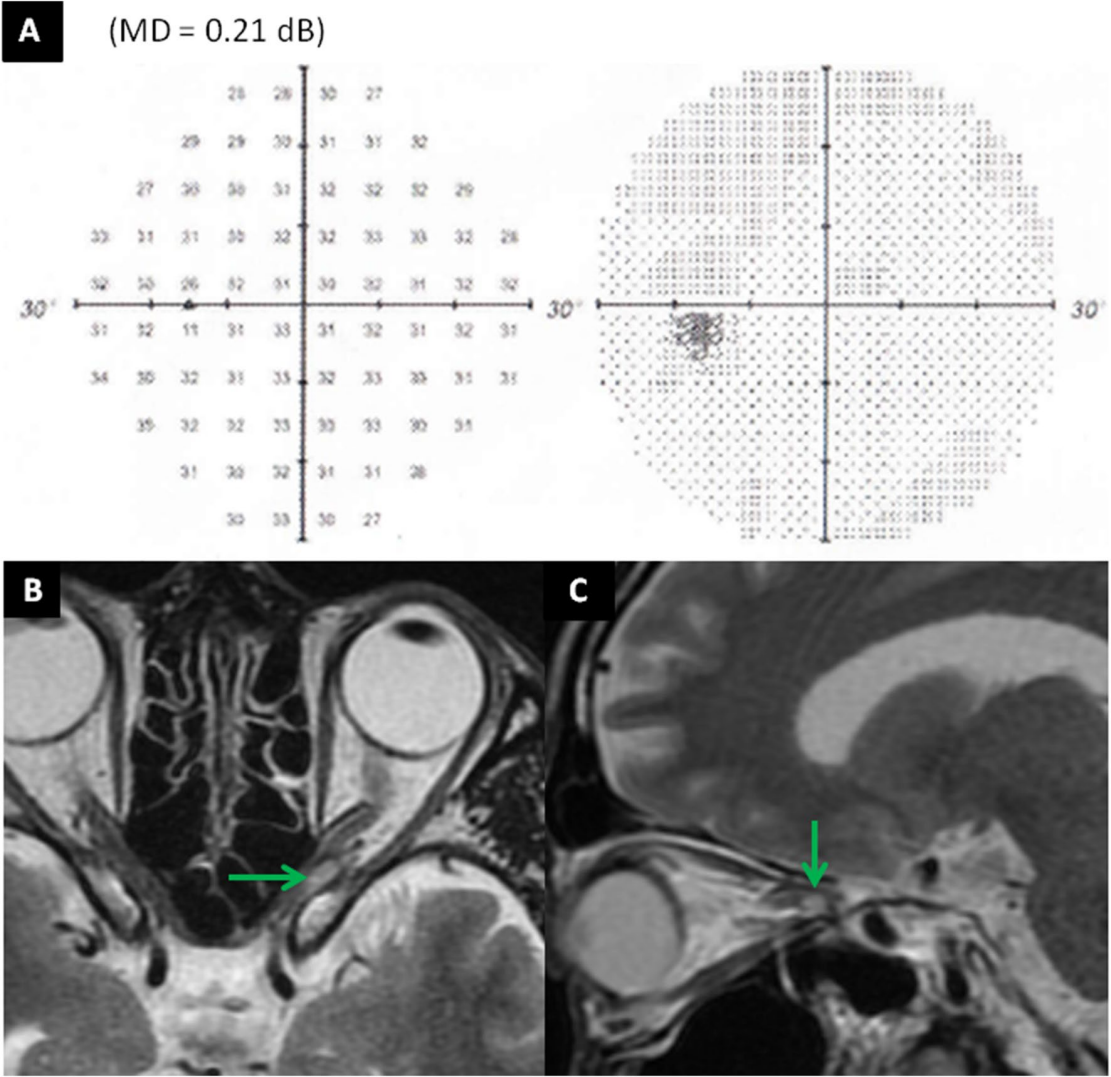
Humphrey automated perimetry showed complete disappearance of the visual field defect (a). Magnetic resonance imaging showed partial regression of the tumor (green arrow), with post-treatment dimensions of 6.5 × 3.5 × 5 mm (volume 0.1 cm^3^) 2.5 months after intensity-modulated radiotherapy completion (b, c)
